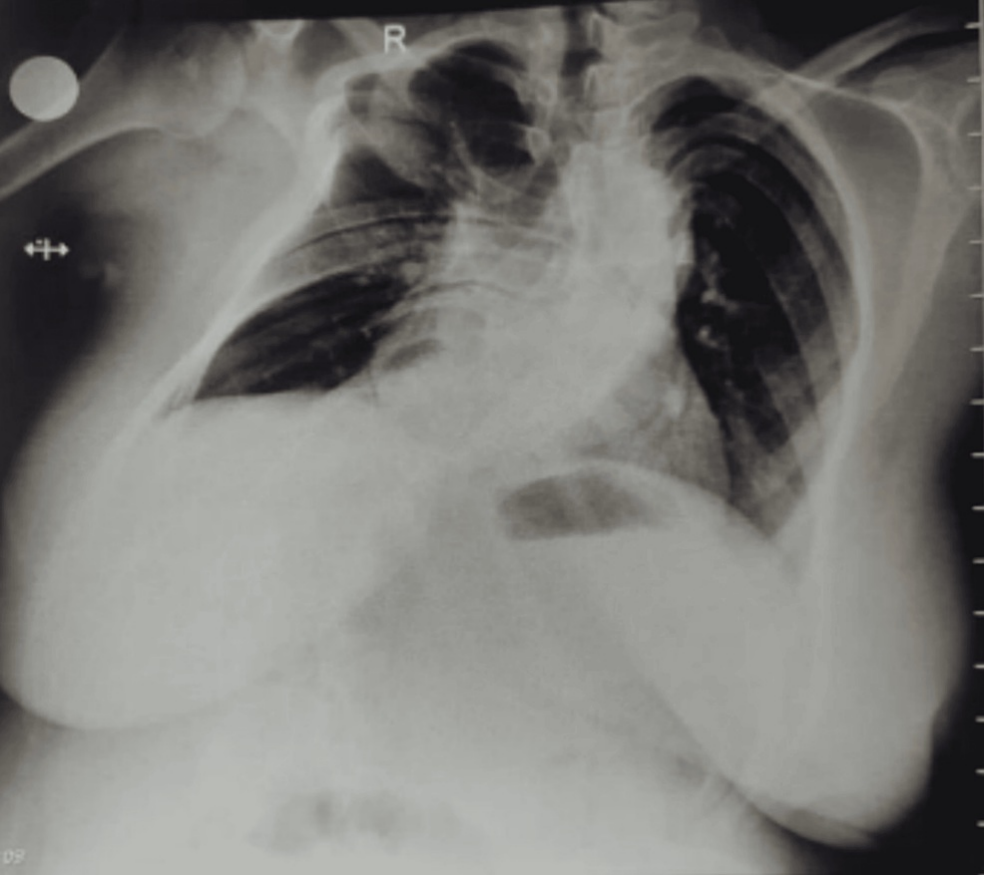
Postoperative posteroanterior chest radiographic view of kyphoscoliotic patient
